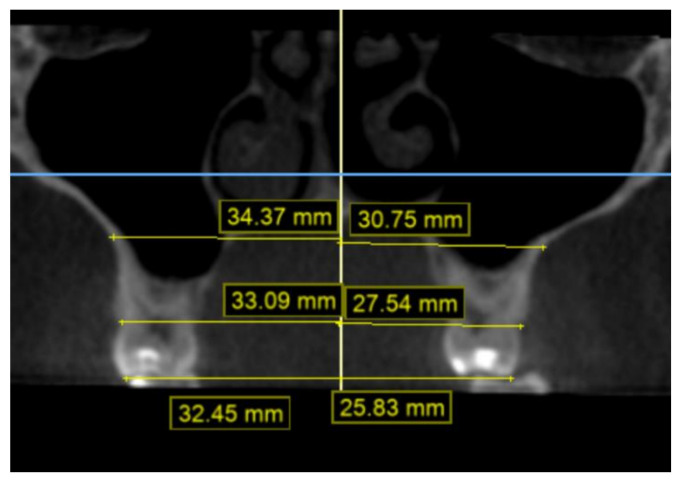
Distances from first molar to median sagittal plane on CBCT scan: Walaridge, groove, and J point.
